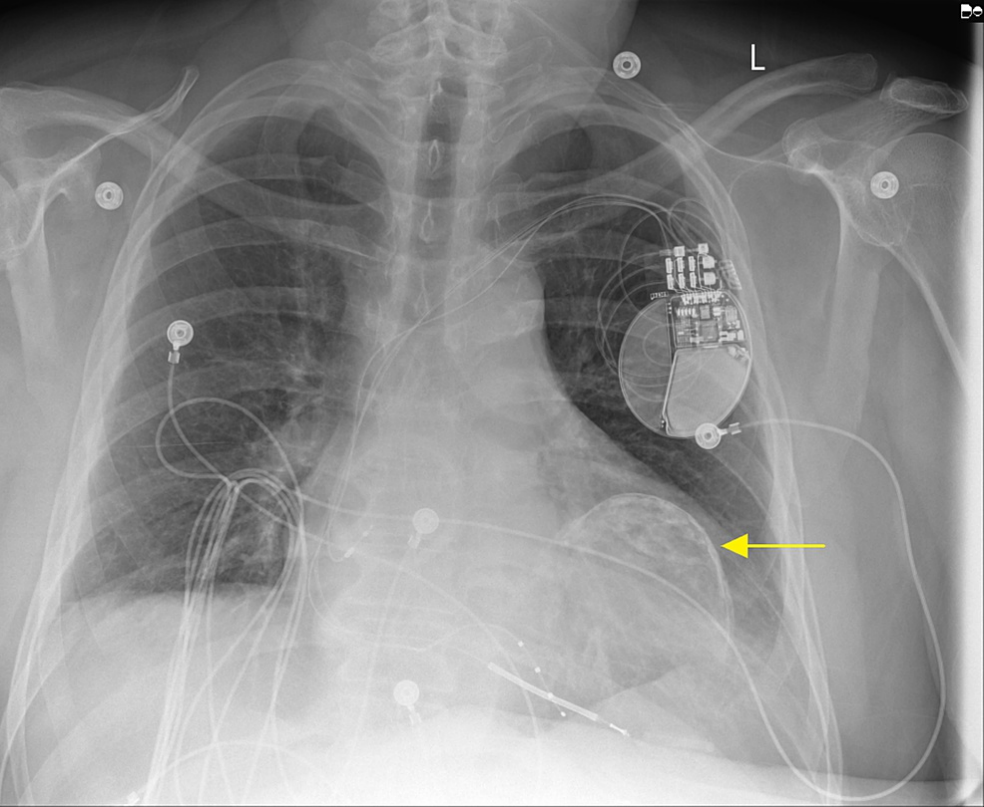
Posteroanterior chest radiograph showing placement of ICD with the calcified ventricular aneurysm wall seen projecting over the left heart (yellow arrow).
